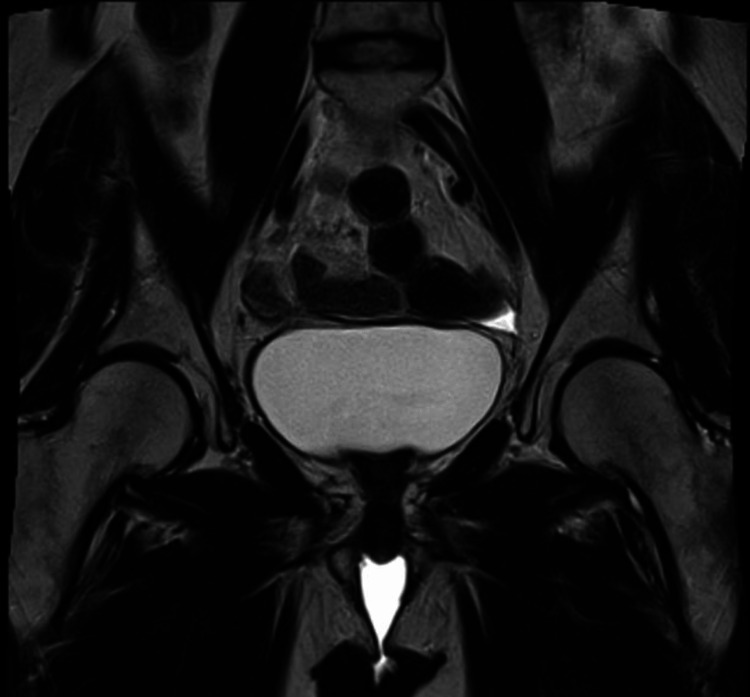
Magnetic resonance imaging showed only cicatricial changes next to the vaginal dome and minimal free liquid in the bottom of the peritoneal sac.
